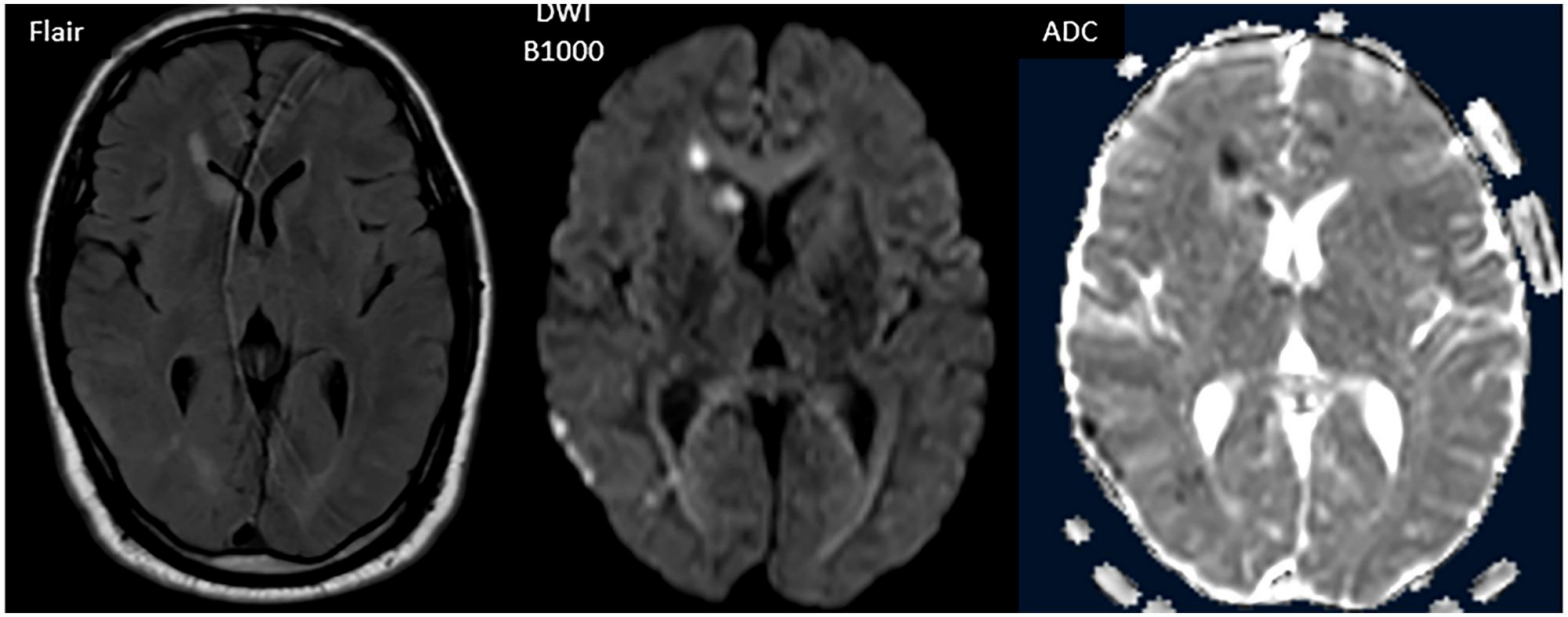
T2-weighted FLAIR, diffusion (B1000) imaging with apparent diffusion coefficient (ADC) mapping, passing through the thalamus and caudate nuclei, revealing FLAIR hyperintensities and diffusion restriction in areas lacking clear delineation, including the head of the caudate nucleus and periventricular white matter. Note: The image quality of the FLAIR sequences is somewhat limited due to patient confusion during the MRI acquisition.
